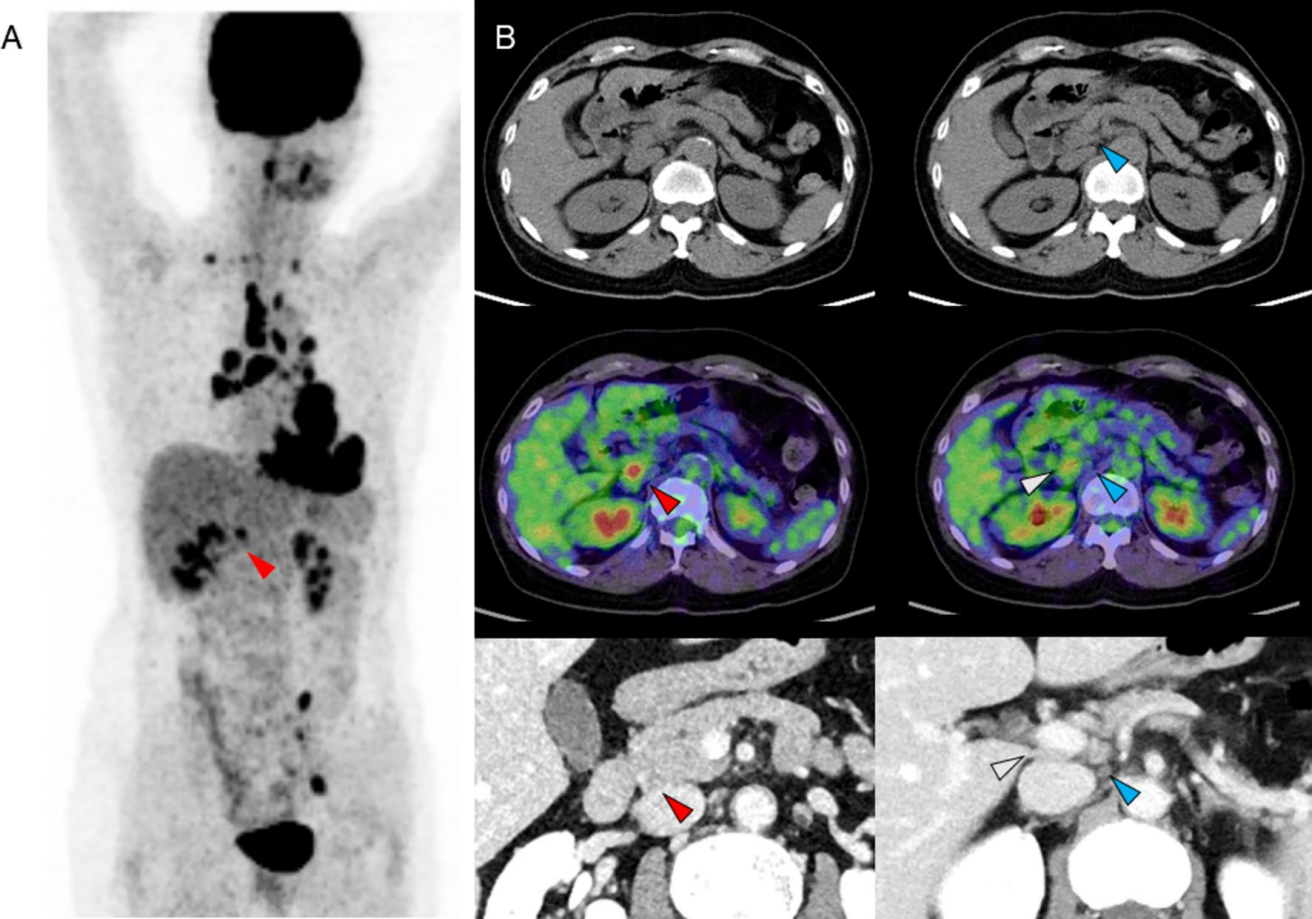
A Maximum intensity projection (MIP) image of FDG-PET, B upper row: CT portion of PET/CT, middle row: fused PET/CT image, lower row: contrast CT image. Focal FDG uptake corresponding to a pancreaticoduodenal lymph node is seen (red arrowhead), identifiable as a small nodule on the CT component of PET/CT, and slightly more conspicuous on contrast-enhanced CT. In contrast, a small periportal lymph node (blue arrowhead) is visualized on contrast-enhanced CT, but shows no FDG uptake. The MIP image also shows intense FDG uptake in the hilar and mediastinal lymph nodes, as well as in the myocardium, suggestive of sarcoidosis involvement
